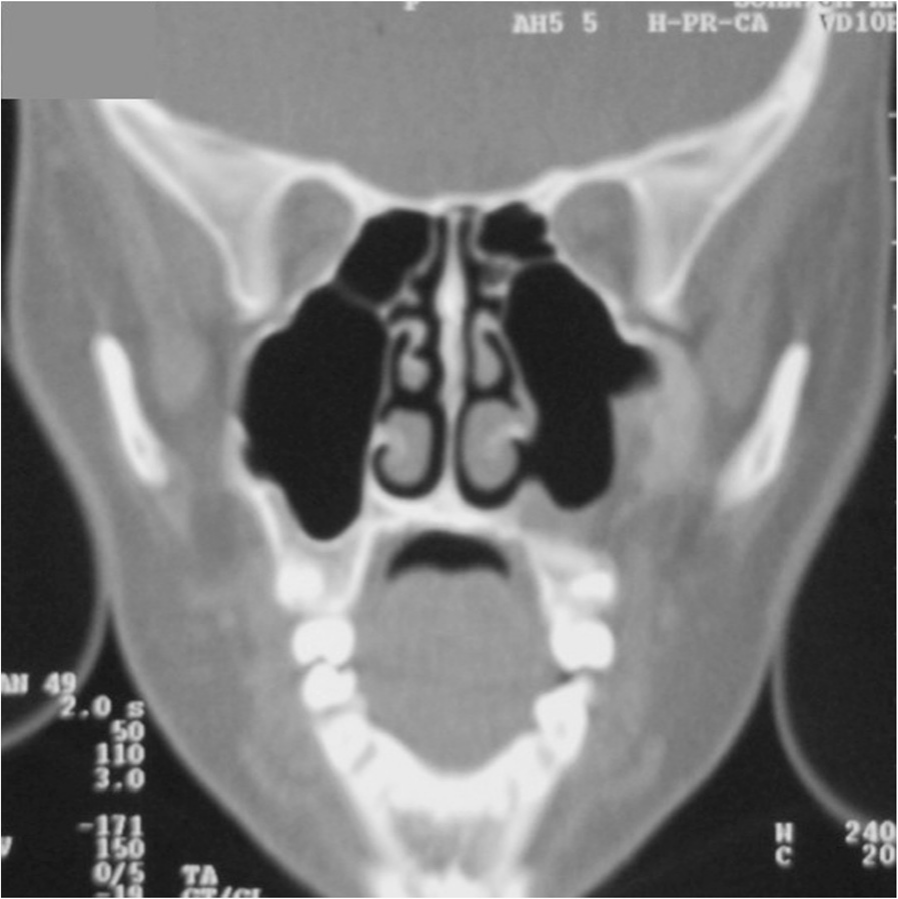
Computed tomography coronal projection at 12 months.
